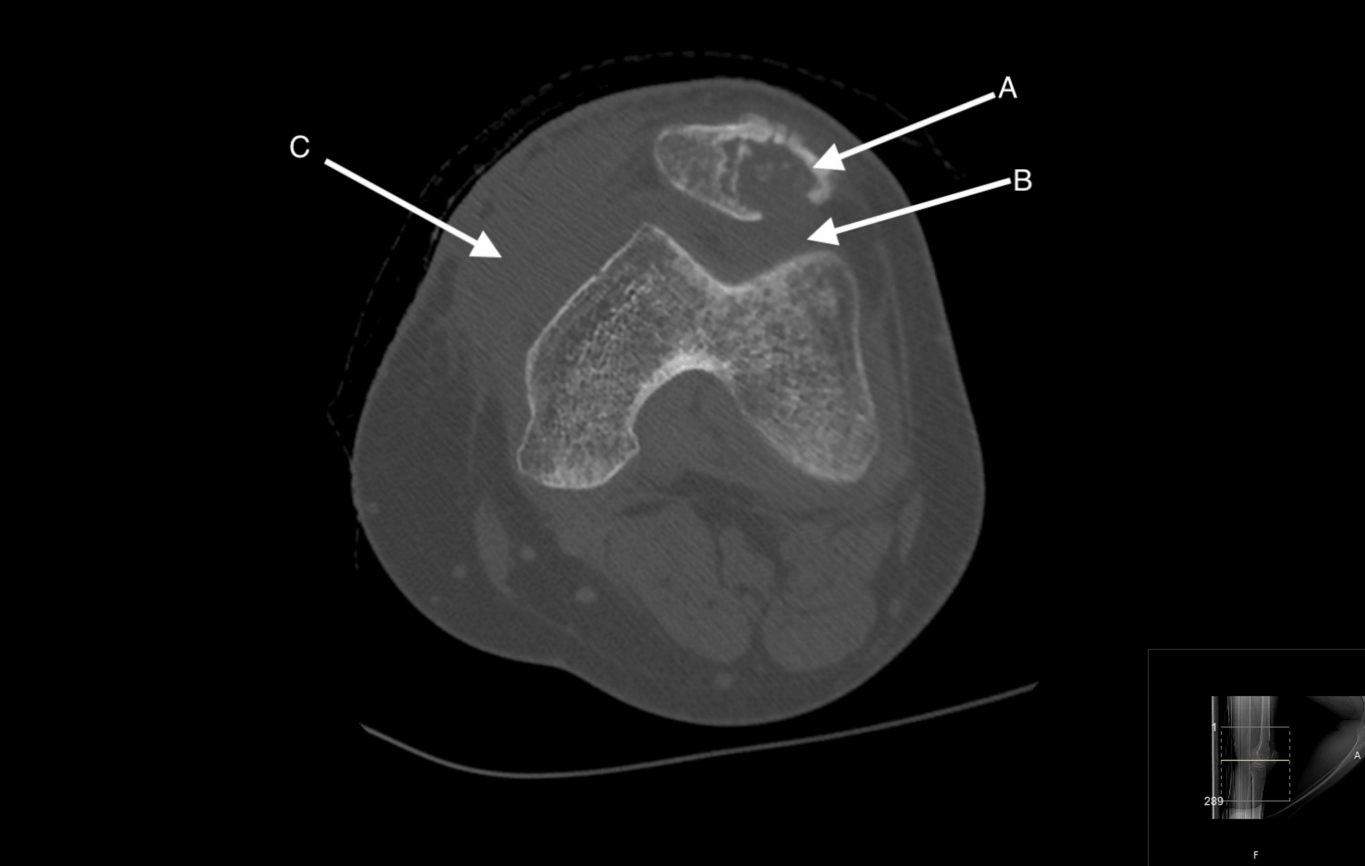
Axial CT scan of the left knee in bone window (A)  Large well-defined lytic lesion (25 x 17 mm) with cortical thinning and scalloping. (B) Extension of this lesion into the infra-patellar fat pad by the formation of a collection. (C) Extra-articular extension of the collection medially and into the subcutaneous soft tissue. The large collection measures 50 x 20 mm
